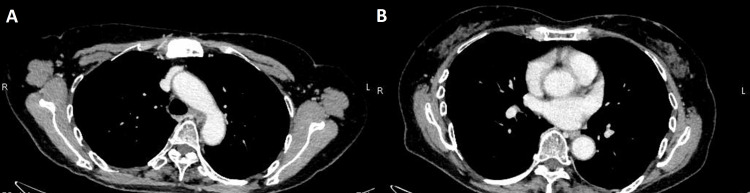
Contrast-enhanced CT six months after discontinuation of pegylated G-CSF showing an improvement in the aortic arch (A) and descending aorta (B). G-CSF: granulocyte colony-stimulating factor
